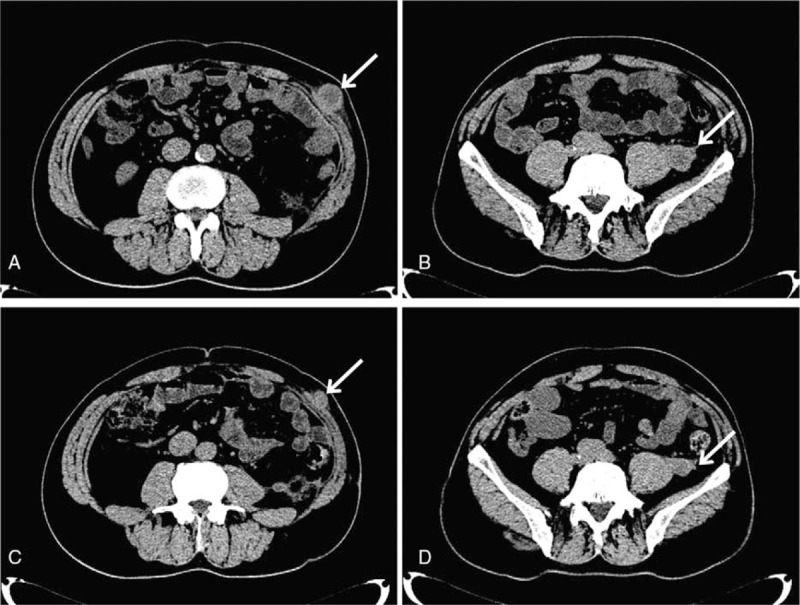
Abdominal CT scanned the left solid abdominal subcutaneous mass and local multiple nodules of the left lateral psoas muscle and the left abdominal wall in 5 months (A, B) and 12 months (C, D) after surgery, respectively. CT = computed tomography.
